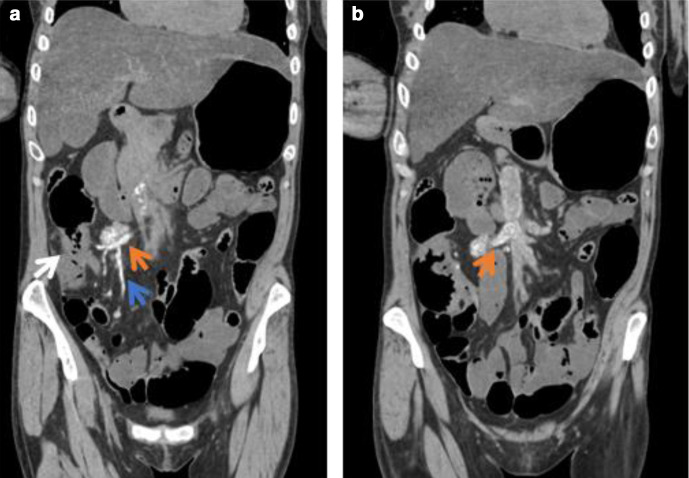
(a, b) Post-mortem CT coronal images (2 months post-CT in Figure 1) showing increased calcification in the SMV tumour thrombus (red arrows) and extending into tributaries (blue arrow). The caecal mass causing near complete luminal obstruction is also noted (white arrow). SMV, Superior mesenteric vein.
